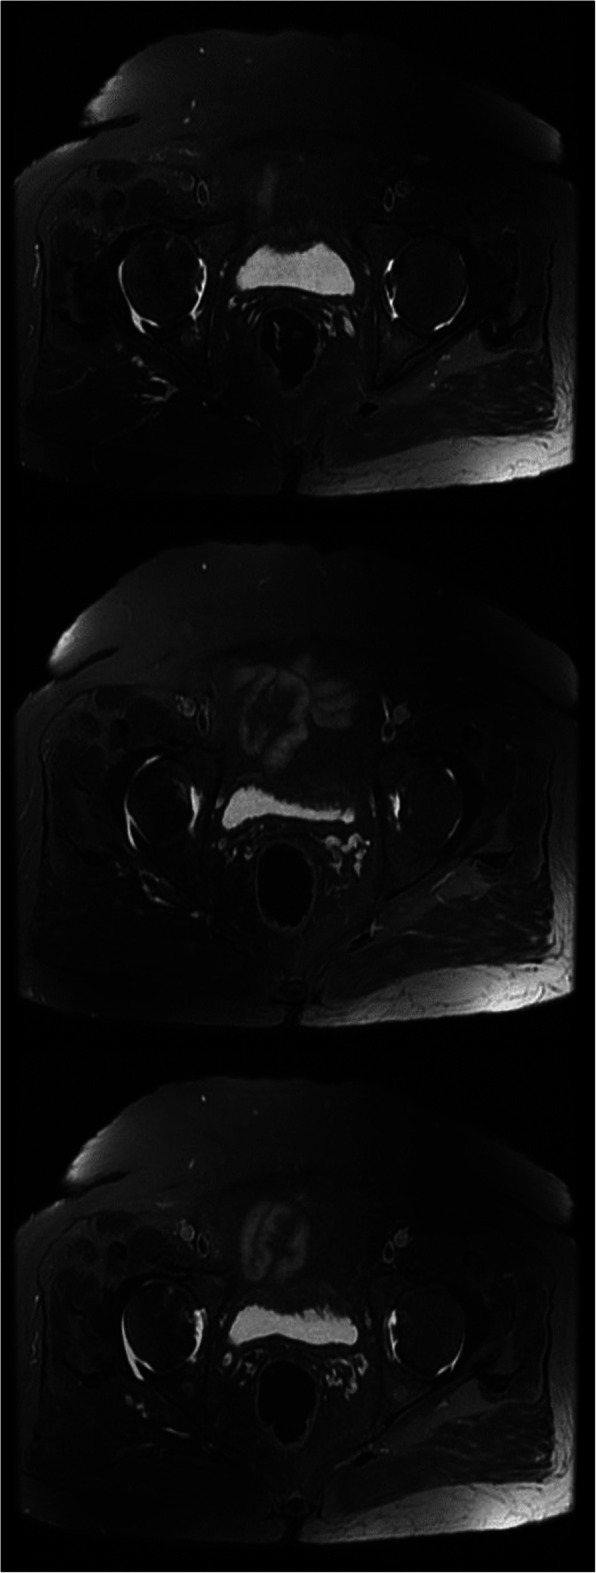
MRI of the pelvis. MRI revealed a fluid rim around the pelvic area, suggesting inflammation in the right hip
